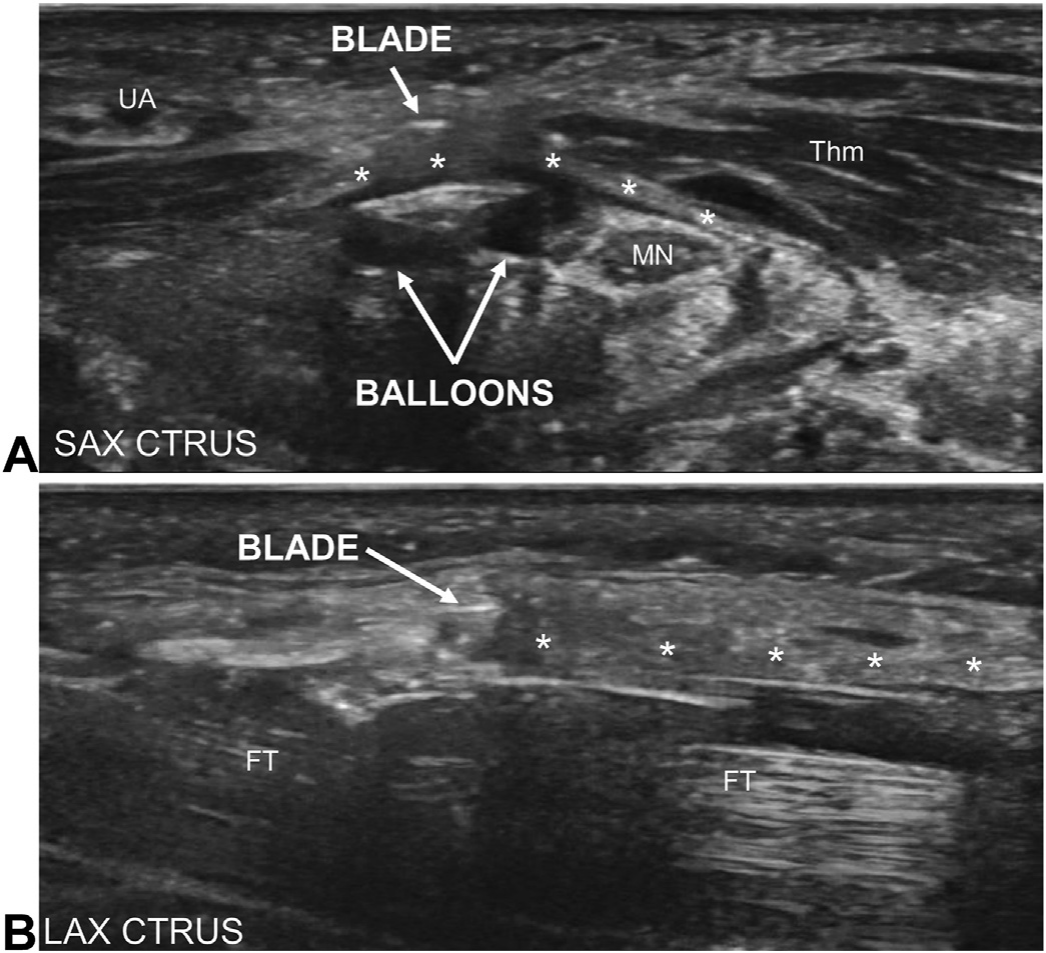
Carpal tunnel release with ultrasound guidance. A Transverse view of the distal carpal tunnel (left = ulnar) showing the blade tip of the device superior to the transverse carpal ligament (TCL, asterisks) and between the ulnar artery (UA) and the median nerve (MN). The balloons were deployed to create space and separate the MN from the centrally located blade. B Longitudinal view (left = distal) showing the device directly under the TCL with the cutting blade visible and engaged to transect the TCL. Thm, thenar muscles.
